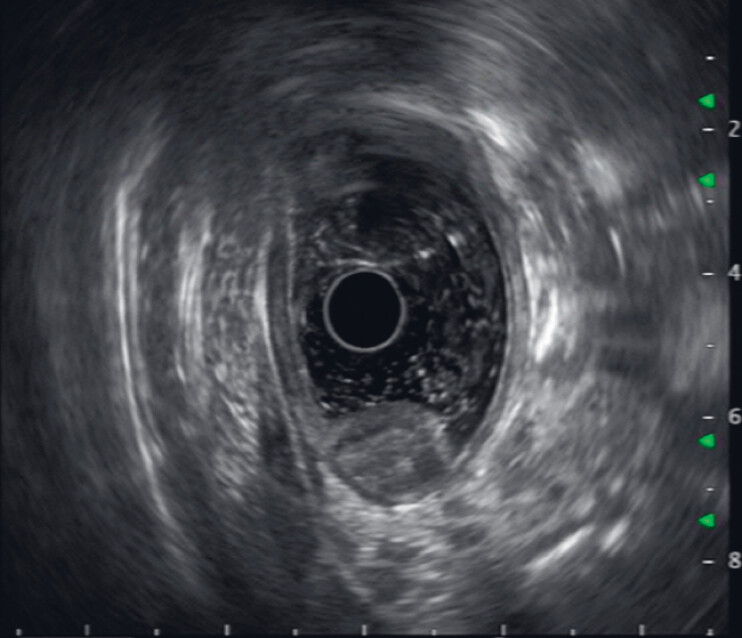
Endoscopic ultrasound view of the lesion showing heterogeneous echogenicity.
